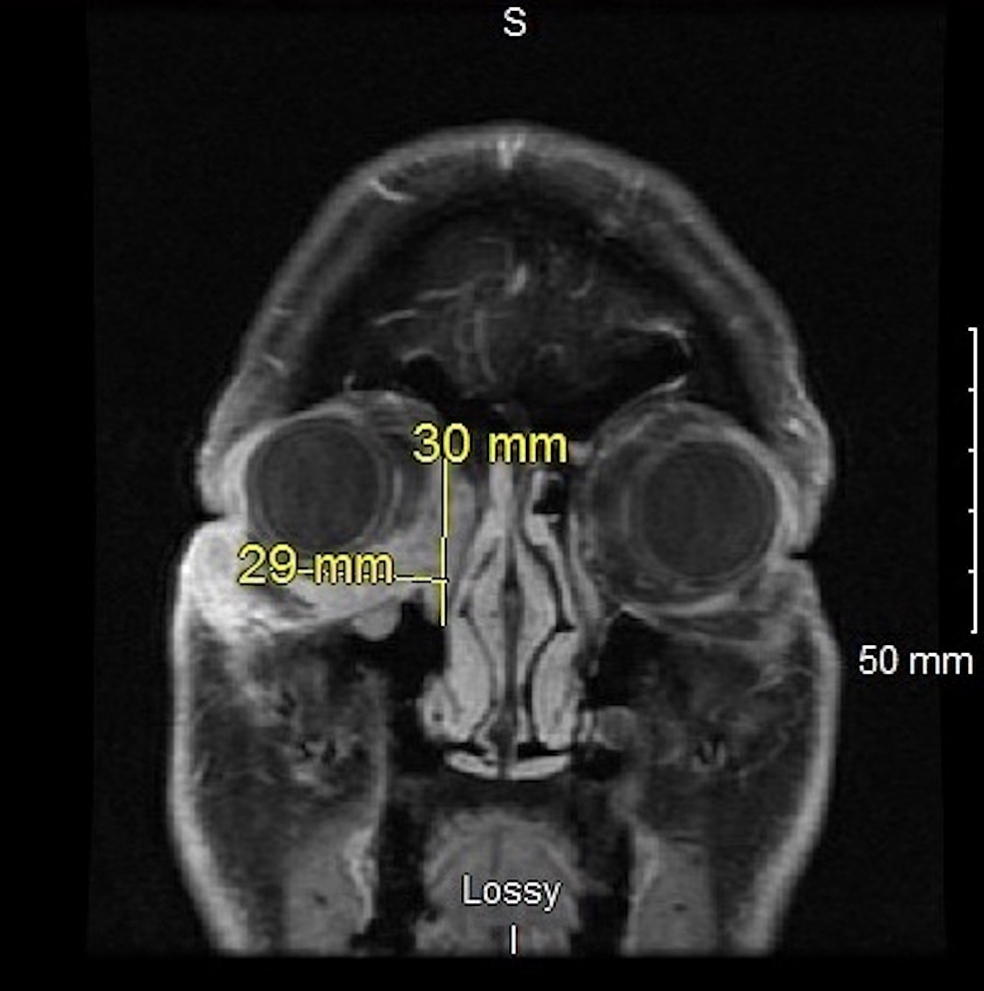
Head MRI coronal section showing the infiltrative mass extending from the inferior right periorbital region to the medial canthus. MRI: magnetic resonance imaging
